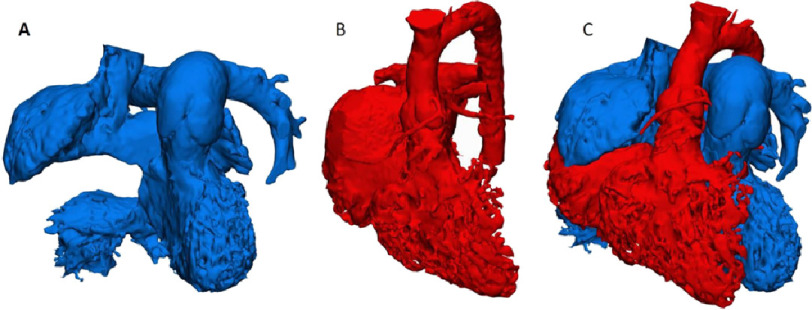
CT derived 3D reconstruction of systemic venous connections (A), pulmonary venous connections (B), combined systemic and pulmonary venous connections (C).
